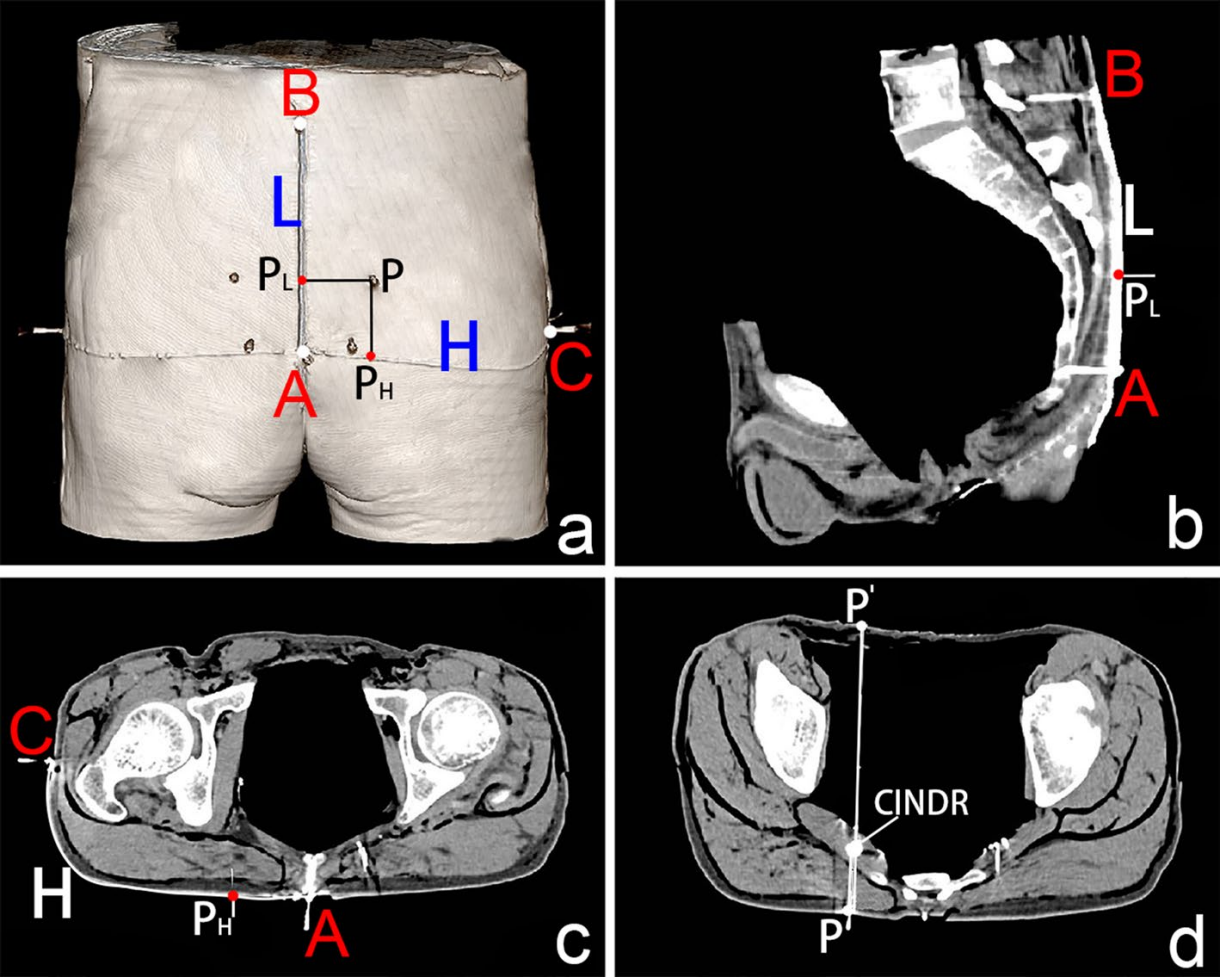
Spiral CT localization image of right piriformis muscle CINDR (male). a Body surface projection position of the CINDR and its design reference line. A = apex of the coccyx, B = spinous process of the 4th lumbar vertebra, C = greater trochanter, and P = surface projection point of CINDR on the buttocks. P_H_ point is the intersection point of the vertical line passing through point P and line H, P_L_ point = intersection point of the horizontal line passing through point P and line L, A-P_H_ = H′, A-P_L_ = L′. b Measurement of the length of the L and L′ lines on the sagittal section of the fourth lumbar vertebra and coccyx apex. c Measurement of the length of the H and H′ lines on the cross-section connecting the tip of the coccyx and the greater trochanter. d Measurement of the depth of CINDR on the cross-section through P. The P′ point is the point where the P point passes through the CINDR point and projects onto the opposite side surface
